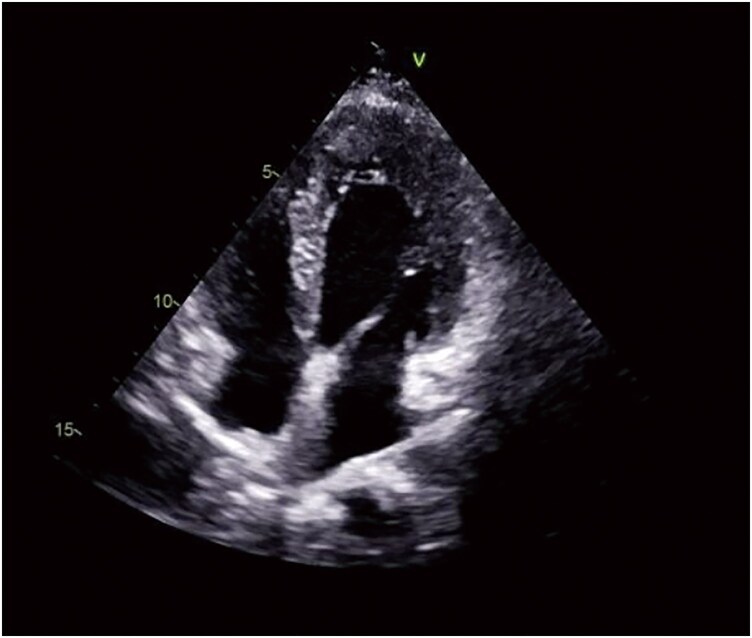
Echocardiography in the apical four-chamber view.
